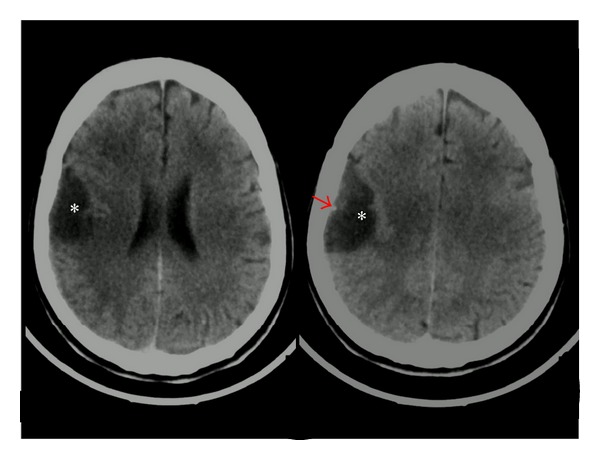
CT scan of the brain. A hypodense, extra-axial image is observed (asterisk), in the right frontoparietal region which imprints over the adjacent brain parenchyma. Lesion hypodensity is similar to the cerebrospinal fluid. A small mural hyperdense nodule (red arrow) is also identified.
